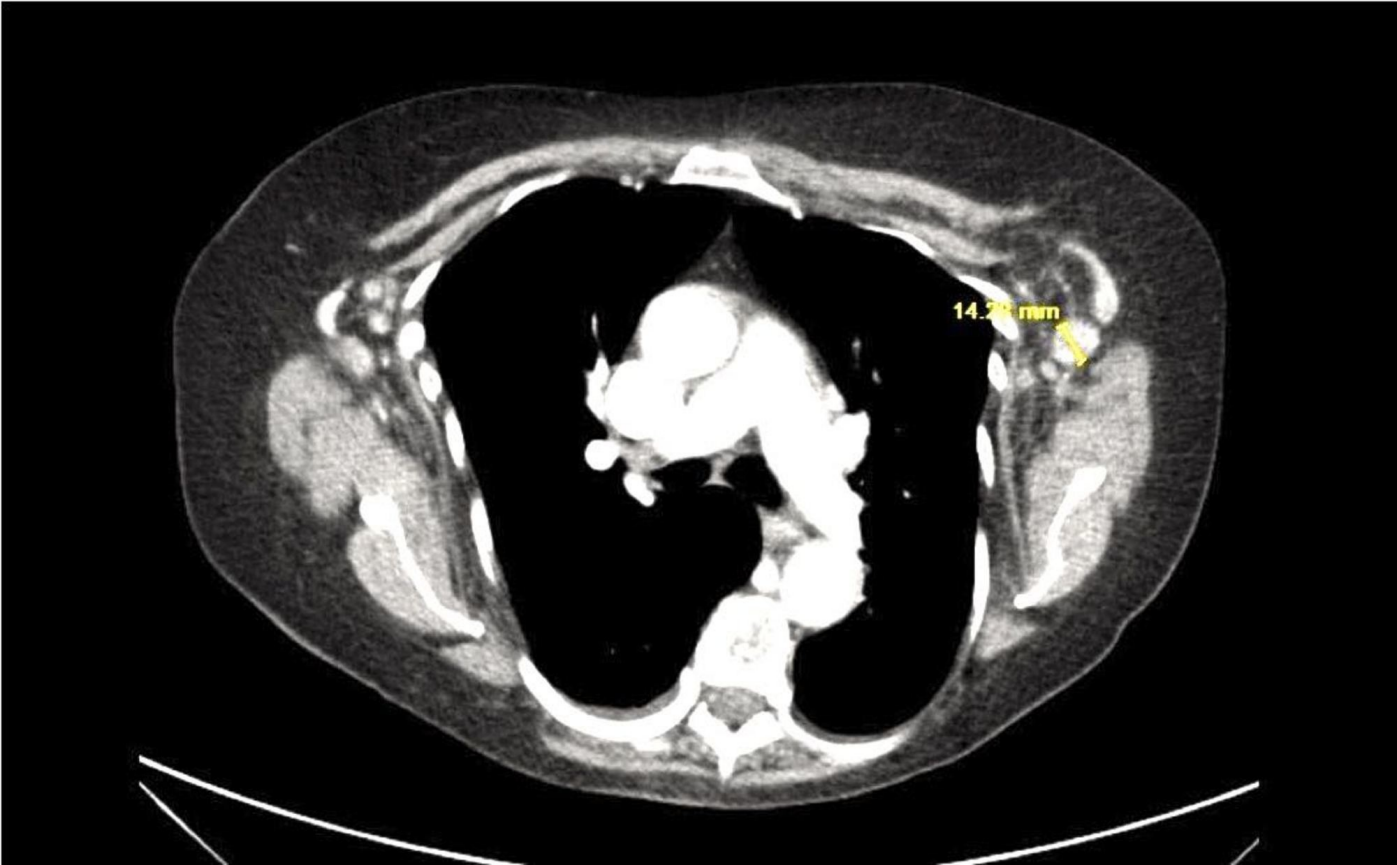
Chest CT showing bilateral axillary lymphadenopathy and enlarged retroperitoneal lymph nodes CT: computed tomography
